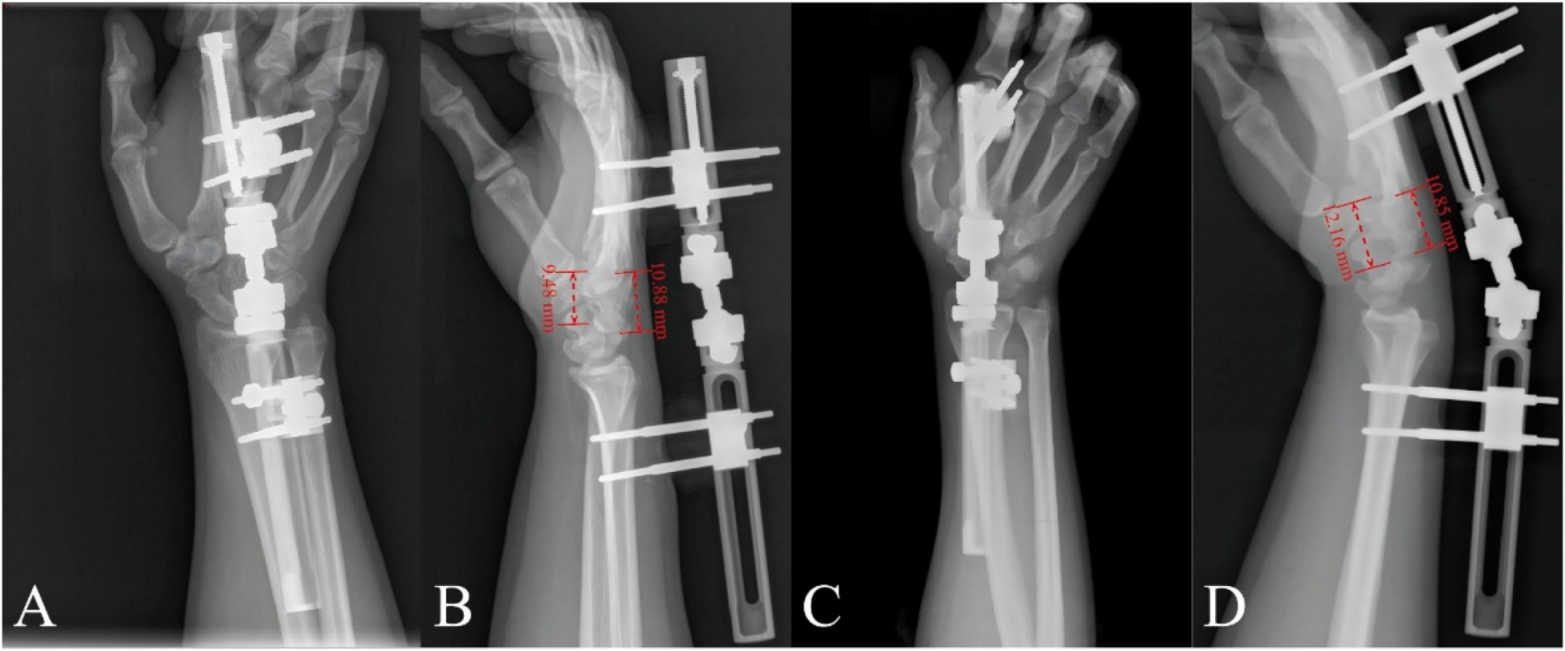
Posteroanterior radiograph (A) and lateral view (B) during stage 1. posteroanterior radiograph (C) and lateral view (D) after 3 weeks of distraction. The measured distance in red represents the lunate–metacarpal distance and capitate height.
